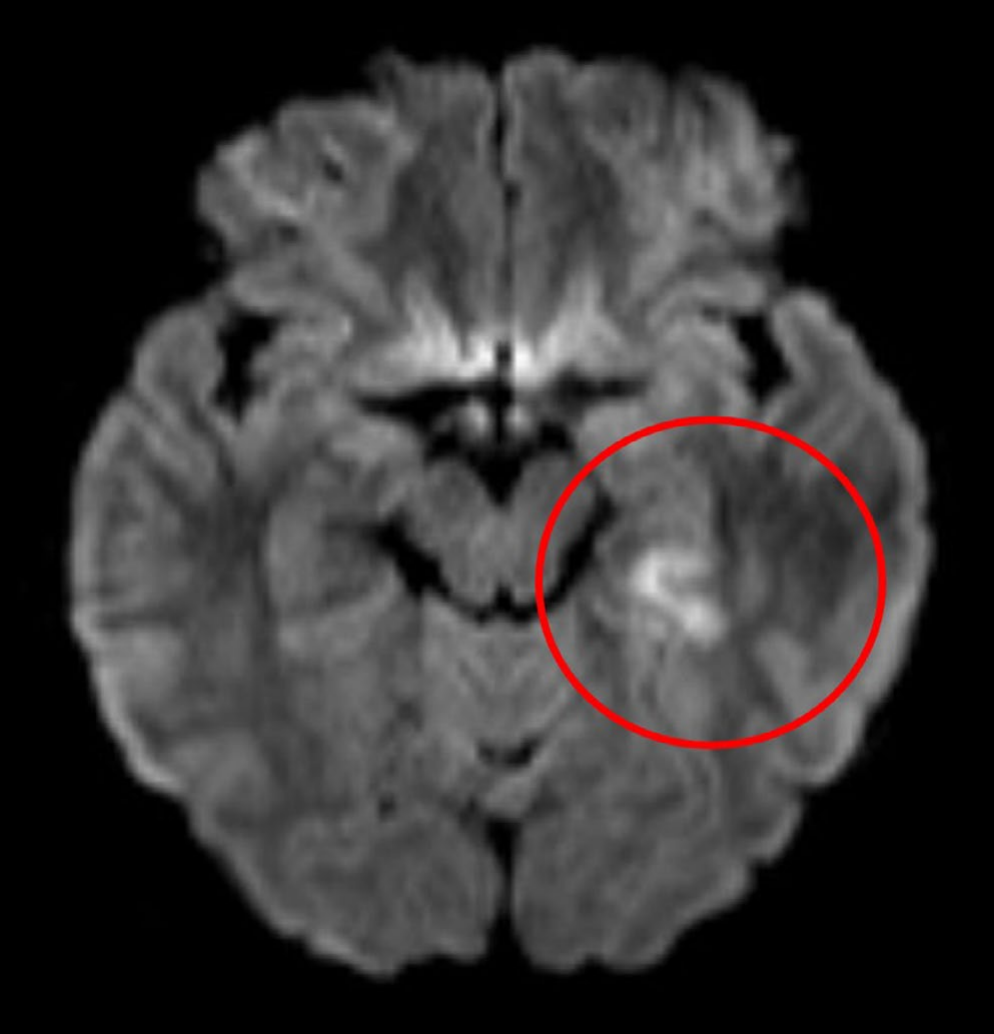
Diffusion‐weighted imaging of brain magnetic resonance imaging showed high signal in left backward lobus temporalis, which indicated fresh cerebral infarction (inside red circle)
